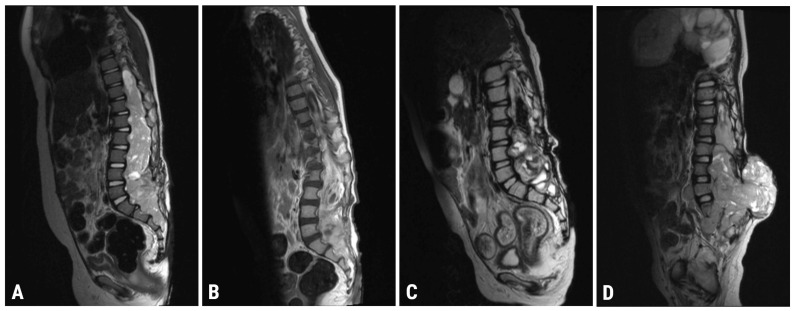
MRI images of the spinal cord in the sagittal projection, T2 weighted: (A) before starting chemotherapy; (B) before starting radiation therapy; (C) immediately after completion of chemotherapy and radiotherapy; (D) 6 months after completion of chemotherapy and radiotherapy.
